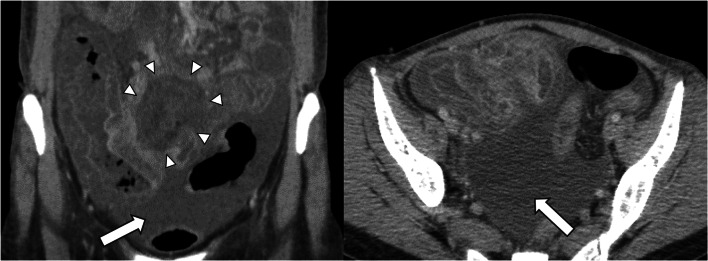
Abdominal contrast-enhanced computed tomography (CT). CT shows marked wall thickening from the ileum to the ascending colon, with massive ascites (arrow) and a low-density area suspicious for an abscess (arrowhead). The appendix was not contrasted and could not be recognized
